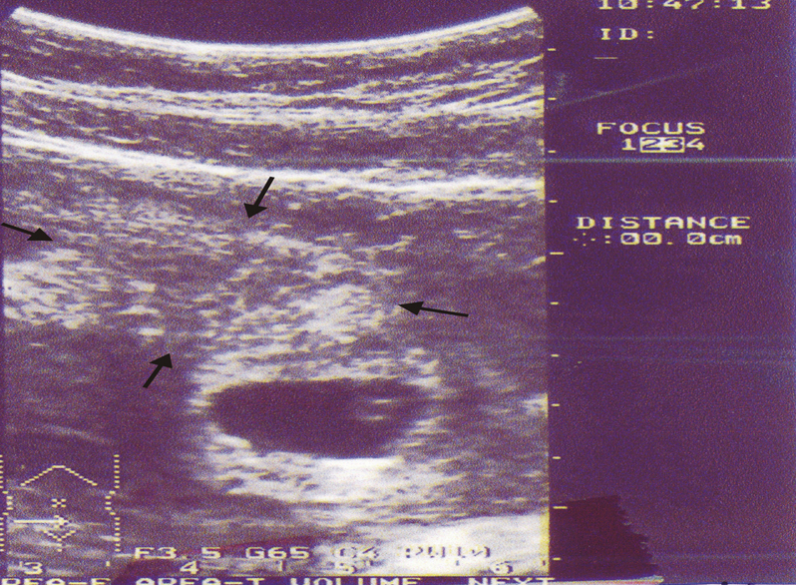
Abdominal sonogram before operation shows intrauterine gestational sac with Yolk sac and an echo complex mass in the left site of the pelvis (arrows).
